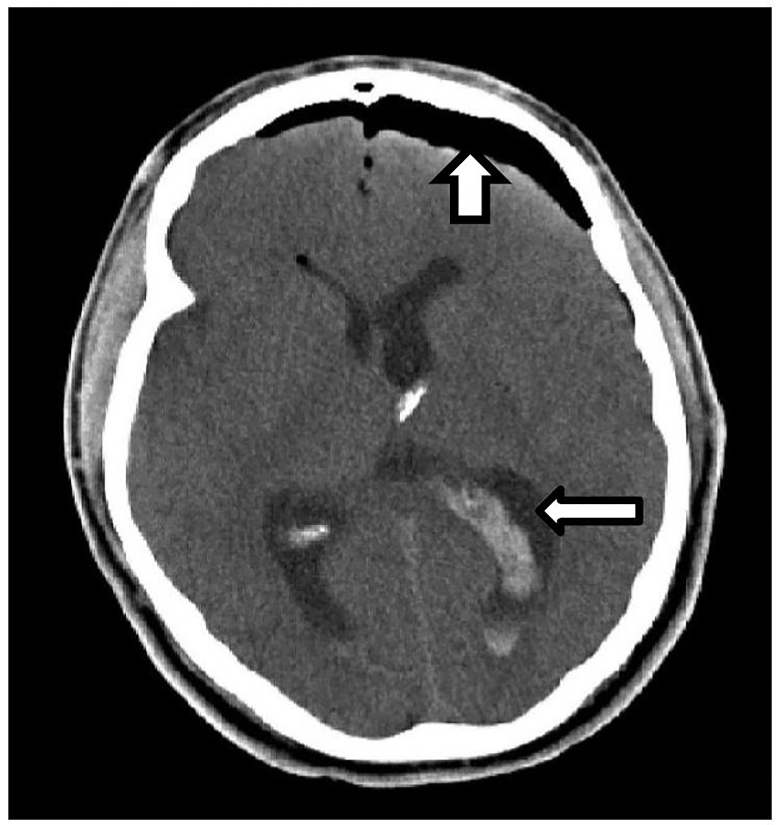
Postoperative noncontrast computed tomography head with presence of pneumocephalus (thick arrow) and intraventricular hemorrhage (thin arrow).
